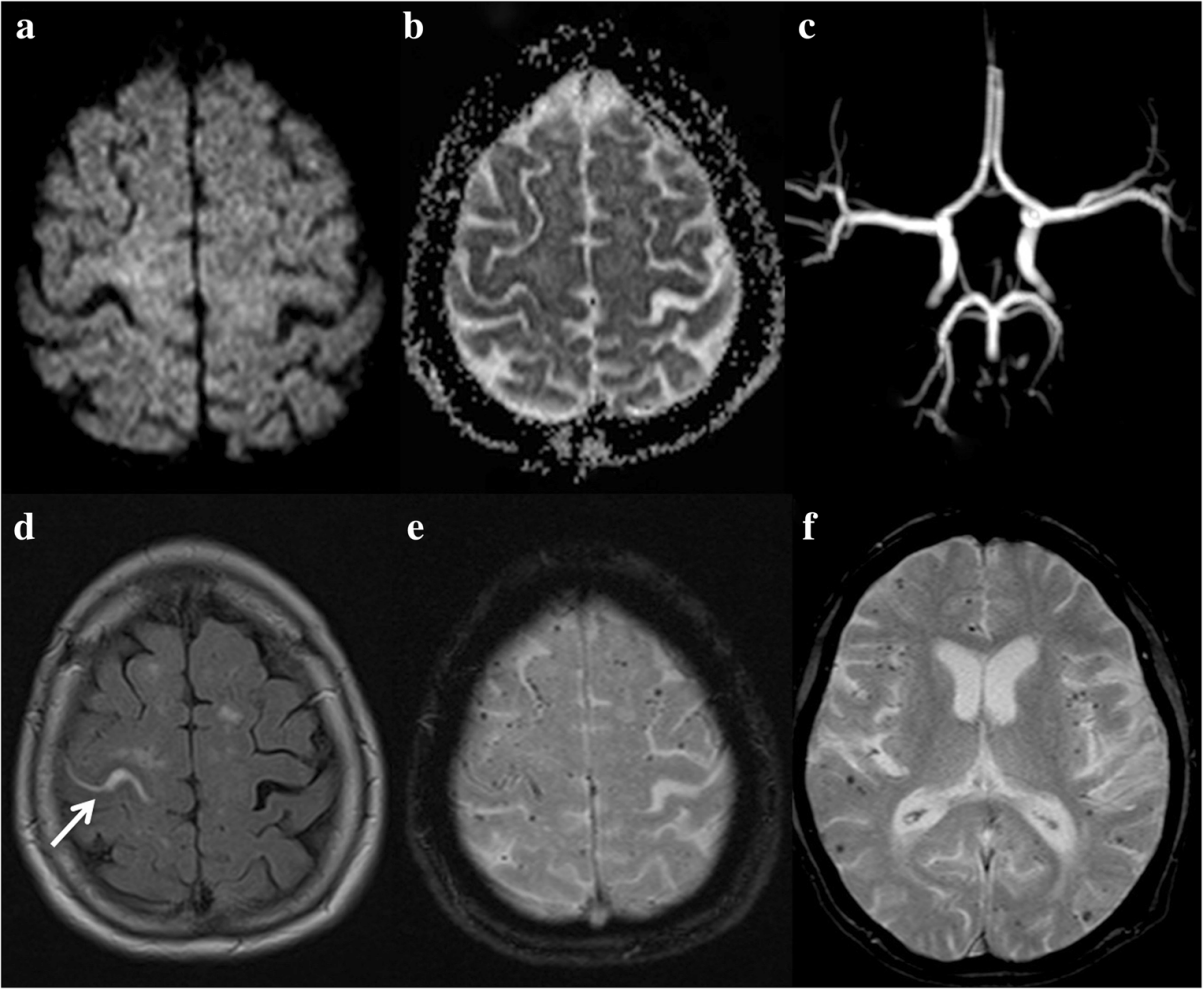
A 68-year-old woman with cerebral convexity subarachnoid haemorrhage (cSAH) in cerebral amyloid angiopathy (CAA) presenting with paraesthesia of the left upper extremity and of the left side of the face. DWI shows no hyperintensity (a) and no restriction (b). No arterial occlusion (c). FLAIR shows hyperintensity in cortical sulcus (d, arrow). T2-GRE shows microbleeds that spared the basal ganglia (e, f)
